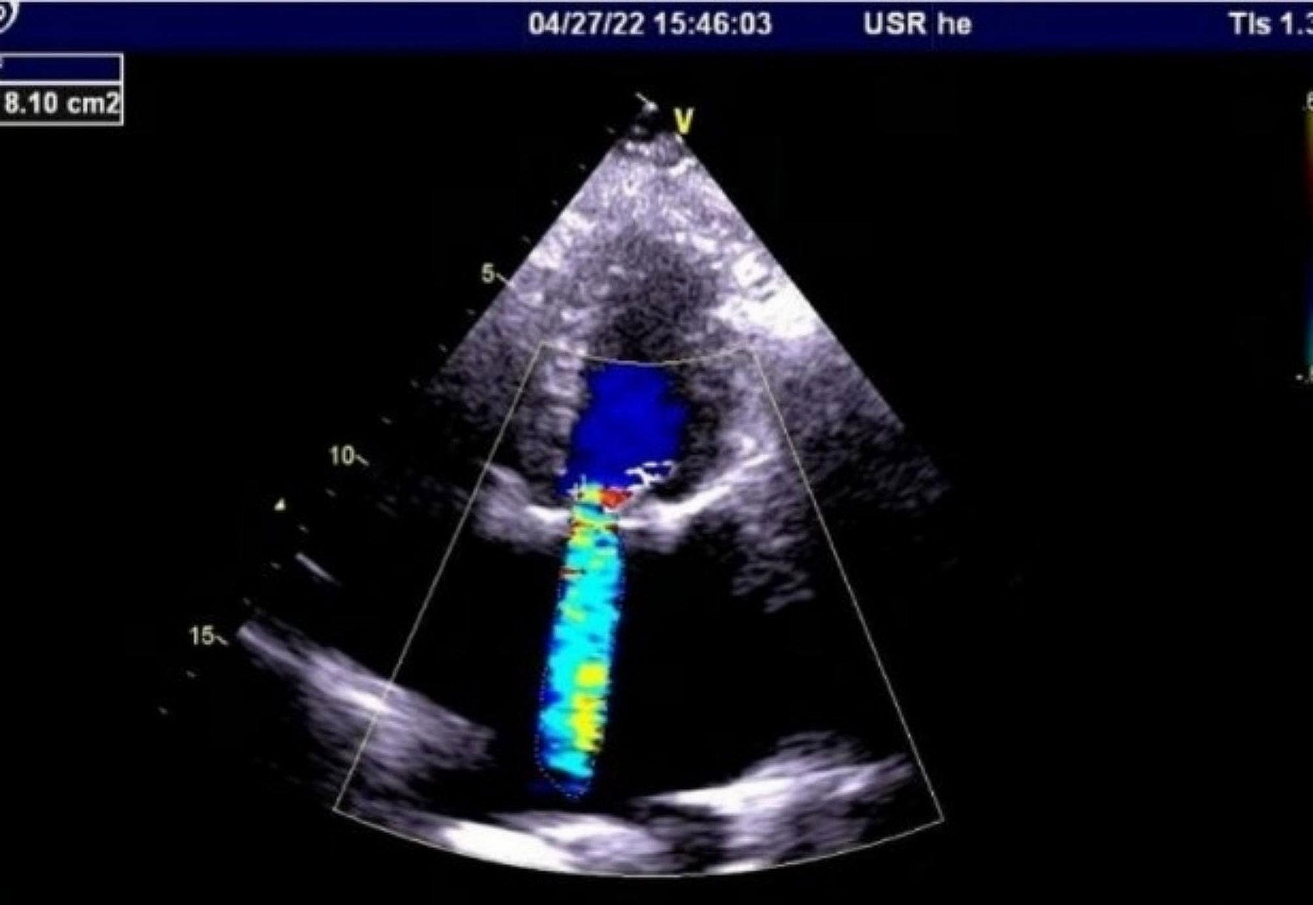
Preoperative transthoracic echocardiogram: The top left corner shows the measured reflux area of 8.1cm2
